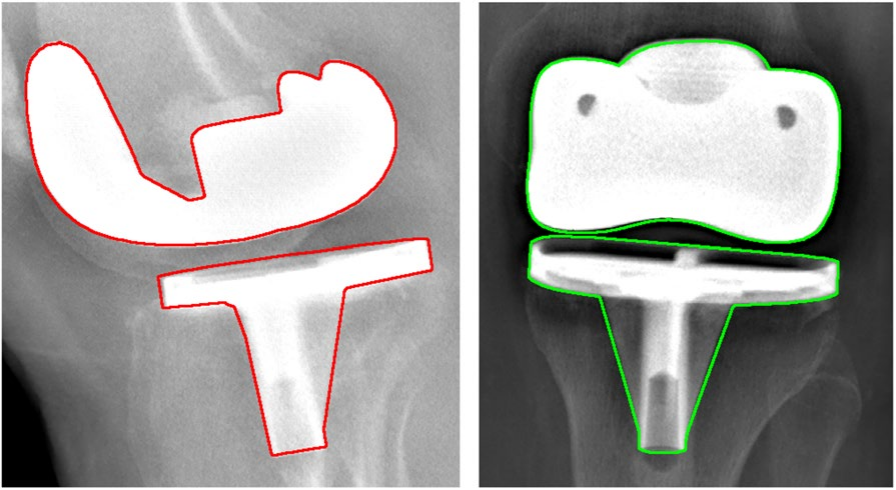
Example of our registration for femoral and tibial components on real radiographic images : lateral image (left) and frontal image (right)
